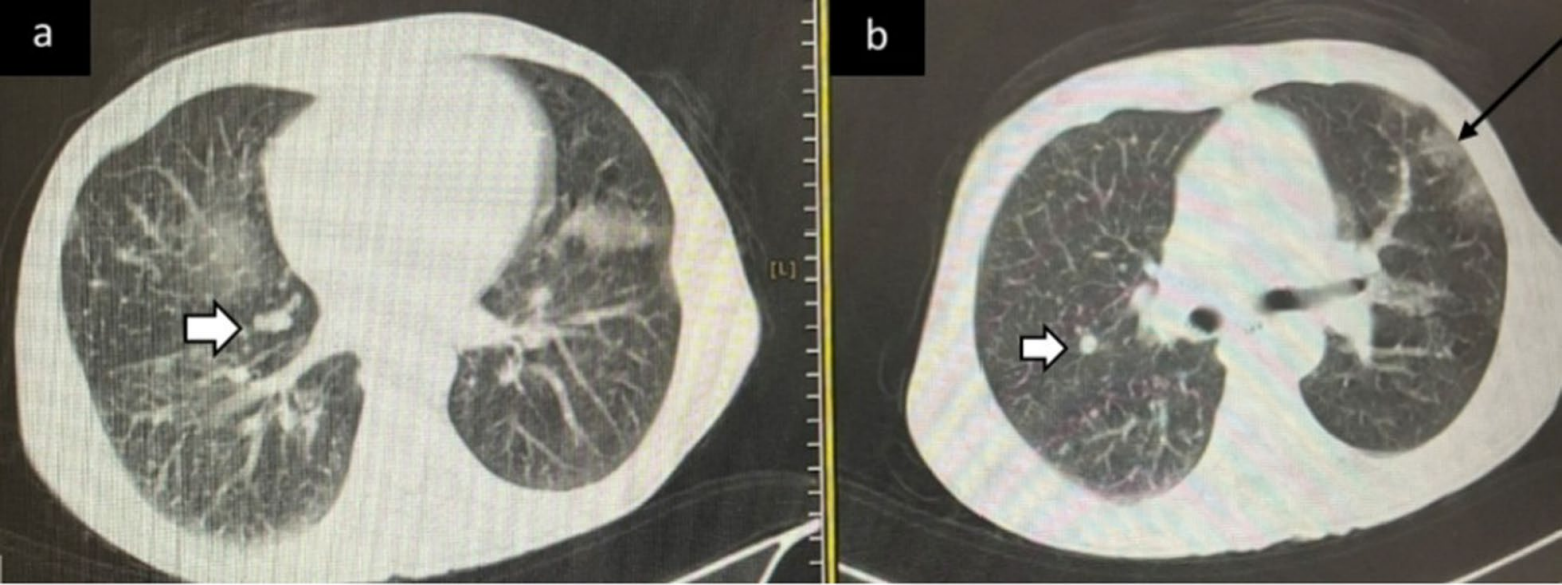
Typical CT imaging features for COVID-19. Unenhanced, axial images of the lungs in a 55-year-old man with hepatocellular carcinoma and positive RT-PCR show peripheral GGO (black arrow, b) with metastatic nodules (white arrows, a, b)
